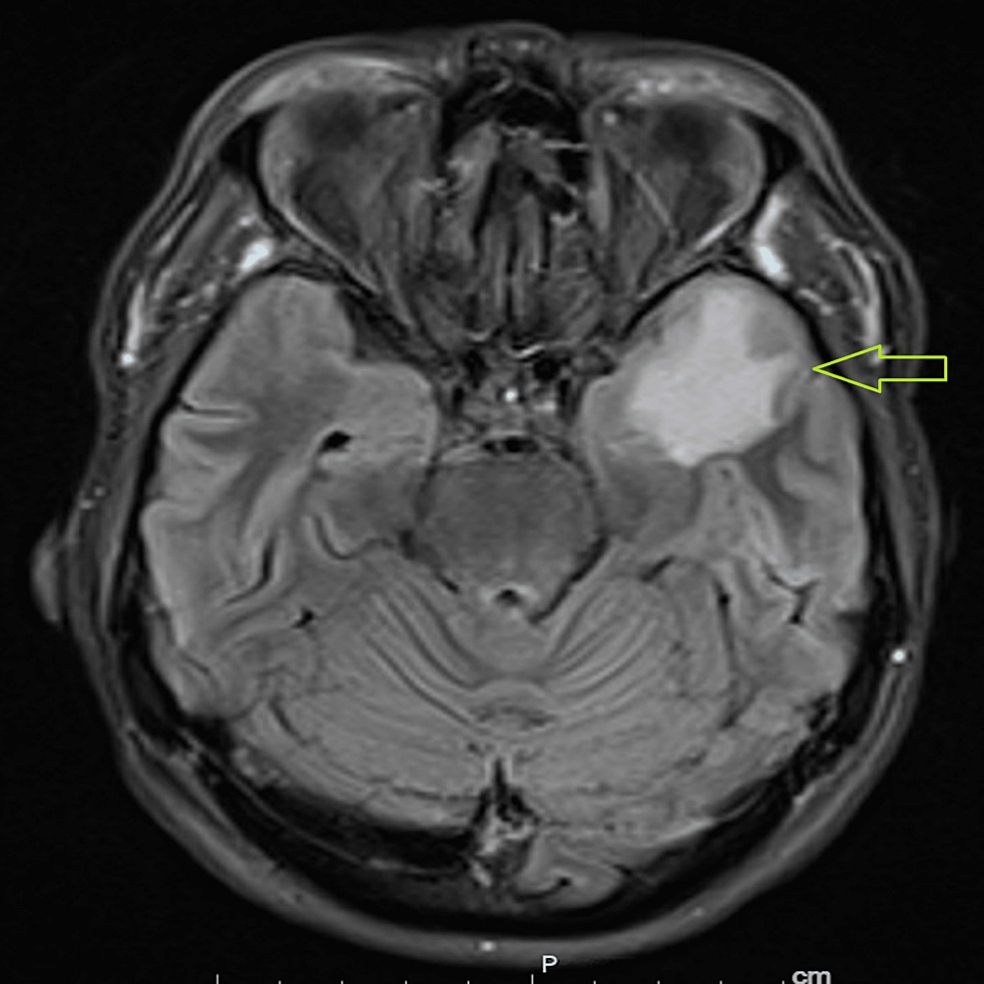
MRI of the brain demonstrating an intra-axial mass in the left temporal pole with associated parenchymal edema
